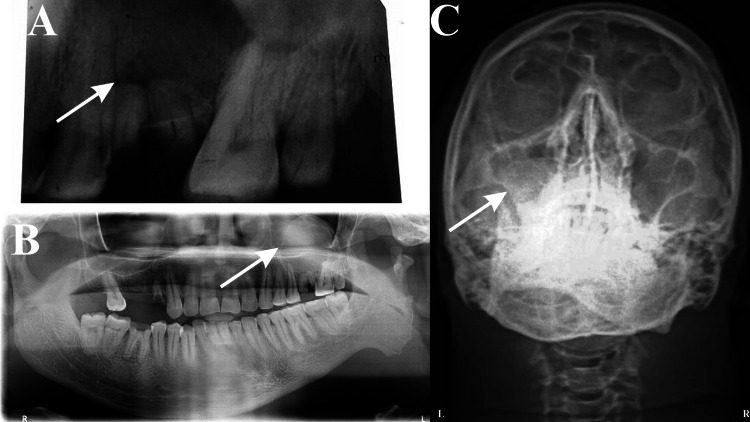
Various radiographic evaluations of the patient (A) Intraoral periapical radiograph showing an ill-defined radiolucency at the apex of tooth 26; (B) Panoramic radiograph demonstrating a hazy, radiopaque lesion occupying the left maxillary sinus; malunion of fractured condylar segments and elongated left coronoid process; (C) Waters' view showing diffuse opacification of the left maxillary sinus
